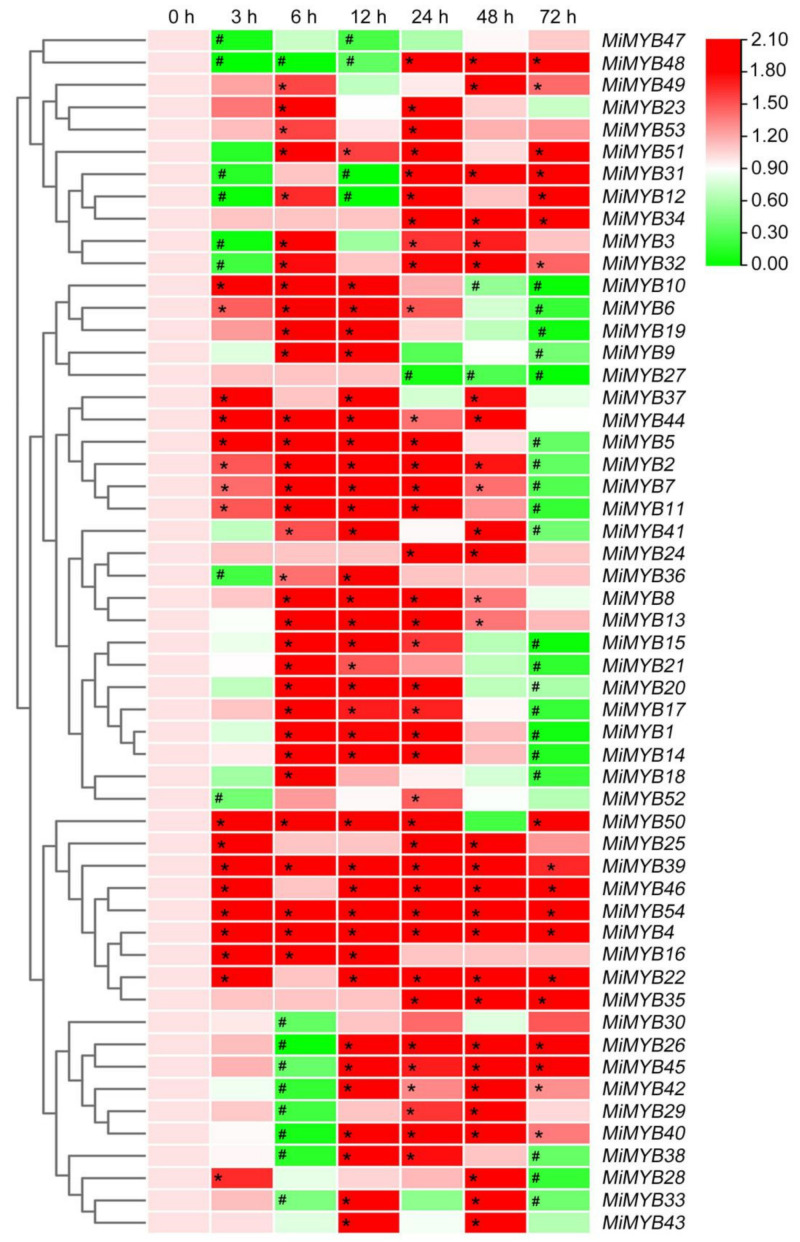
Expression patterns of *MiMYBs* in response to *C*. *gloeosporioides* infection. Relative transcript levels of *MiMYBs* with 2 × 10^6^ conidiospores/mL *C*. *gloeosporioides* infected mango leaves by spray at 0, 3, 6, 12, 24, 48, and 72 h. The relative transcript levels of *MiMYBs* in the non-sprayed mango leaves (0 h) were normalized to 1.0. The color scale represents gene expression levels with high transcript levels (red) or low transcript levels (green). The calculation method was 2^−∆∆CT^, and ‘*’ means the value exceeds 1.5, while ‘#’ means the value is below 0.5.
